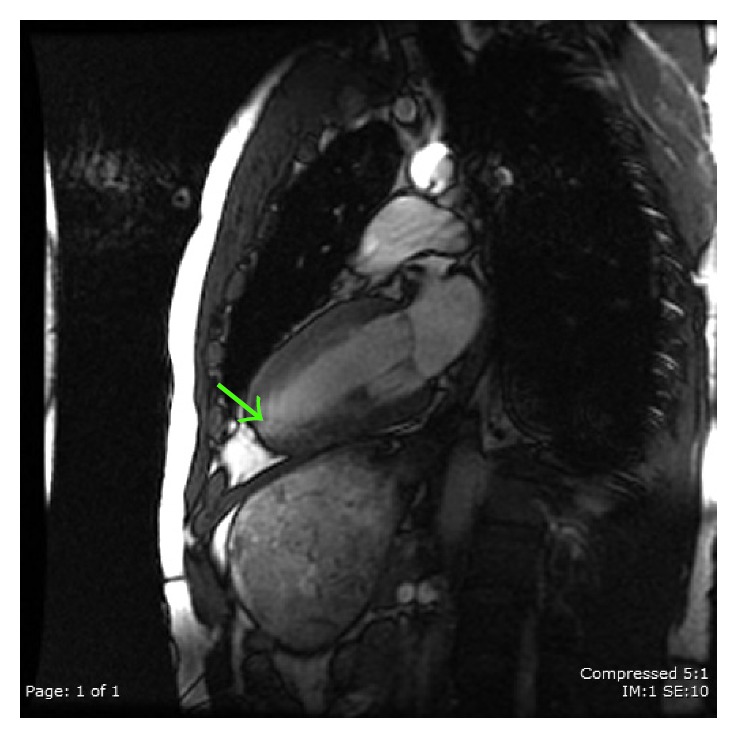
Corresponding T2 images of cardiac MRI 2-chamber view with an apical focal area of high T2 signal intensity in midmyocardium indicating focal edema at the apex.
